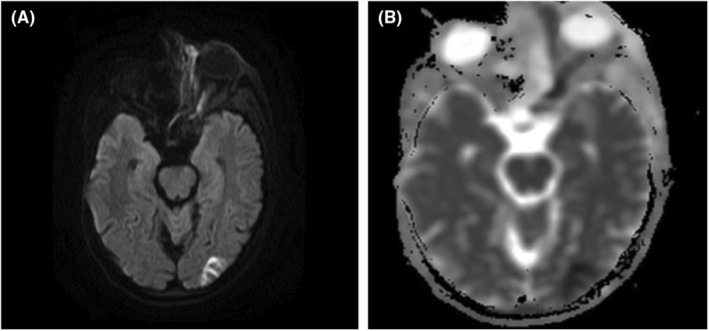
(A, B) Diffusion‐weighted imaging (DWI). (A) shows hyperintense signals within the left optic nerve, which correlates with hypointense signals on apparent diffusion coefficient (ADC) mapping (B), suggesting diffusion restriction consistent with optic nerve infarction. Further diffusion restriction is seen in the left occipital lobe suggesting an intracranial extension of the disease process.
